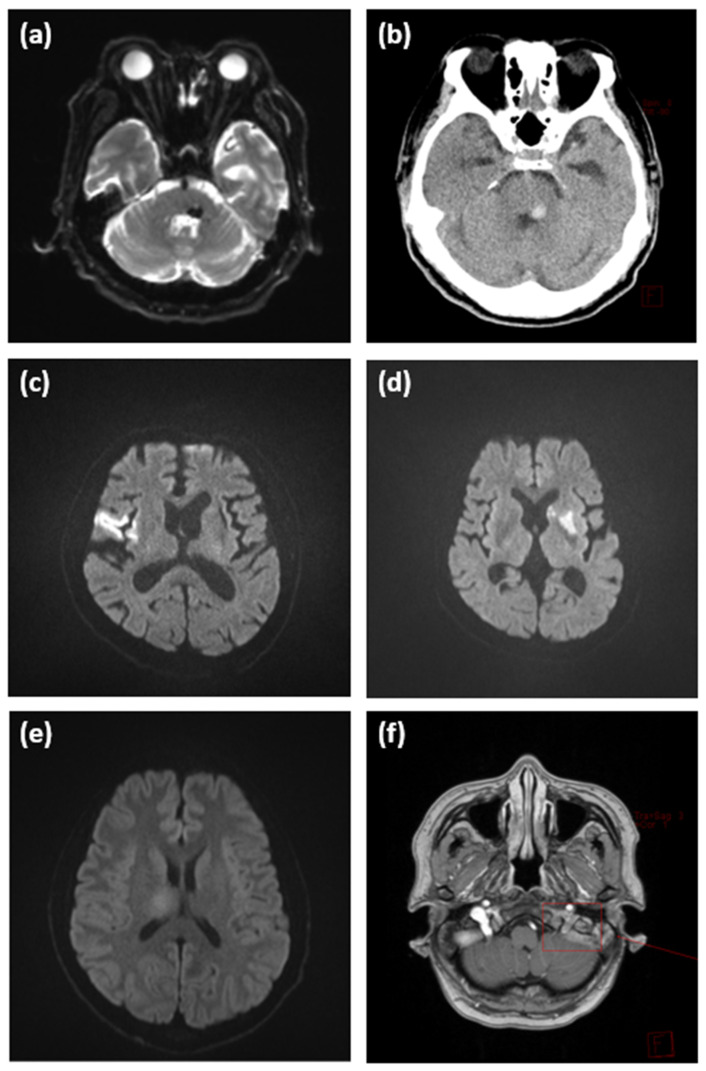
Brain lesions detected in patients presenting with facial palsy. (a) Diffusion-weighted MRI showing acute hemorrhage in the left pons (Case #1). (b) Non-contrast axial CT scan of the brain, obtained after diffusion-weighted MRI (a), with a high-density window setting (WL = 1054, WW = 95), showing a hyperdense pontine lesion consistent with hemorrhage (Case #1). (c) Diffusion-weighted MRI showing acute infarction in the right middle cerebral artery territory (Case #2). (d) Diffusion-weighted MRI showing acute infarction in the left basal ganglia and corona radiata (Case #7). (e) Diffusion-weighted MRI showing a mass-like lesion in the right thalamus, suspected to be a brain tumor (Case #12). (f) Contrast-enhanced 3D T1-weighted axial MRI (gadolinium phase) showing brain metastasis involving the left jugular foramen (Case #9). The red box and arrow indicate the enhancing lesion at the left jugular foramen. The lesion was not clearly visible on diffusion-weighted imaging. MRI—magnetic resonance imaging; CT—computed tomography; MCA—middle cerebral artery.
